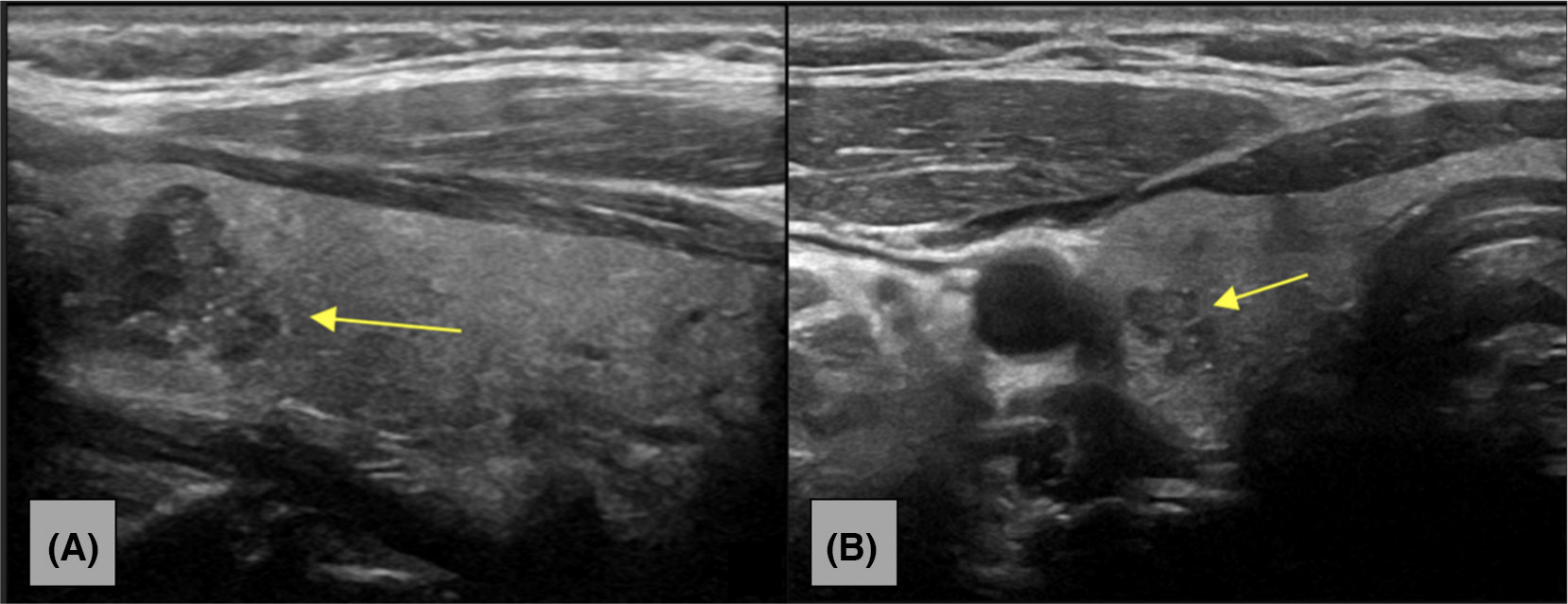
(A) Long‐axis ultrasound image of the right thyroid lobe demonstrates a 1.5 × 1.0 × 1.3 cm hypoechoic wider than tall solid nodule with irregular margins and punctate echogenic foci (yellow arrow). Right thyroid lobe ultrasound in longitudinal (A, B) and transverse (C, D, E) planes demonstrate a 1.5 × 1.0 × 1.3 cm hypoechoic wider than tall solid nodule with irregular margins and punctate echogenic foci (yellow arrow). Mildly increased vascularity is exhibited on color Doppler investigation (B, D). (B) Transverse ultrasound image of the right thyroid lobe demonstrates a 1.5 × 1.0 × 1.3 cm hypoechoic wider than tall solid nodule with irregular margins and punctate echogenic foci (yellow arrow). Keywords: ACR TI‐RADS Criteria.
